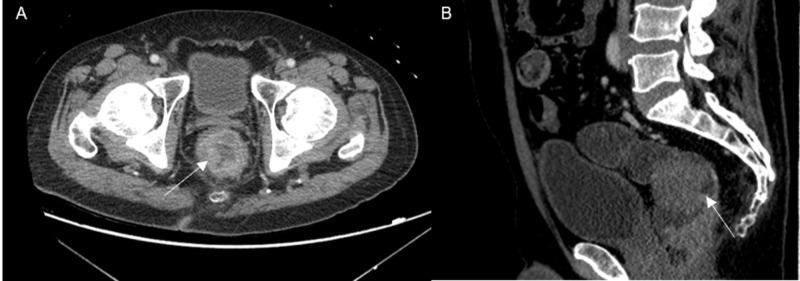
Contrast-enhanced computed tomography (CT) scan of the pelvis Axial (A) and sagittal (B) images from contrast-enhanced CT of the pelvis showed a large rectosigmoid mass (arrow) suggestive of a colorectal malignancy
